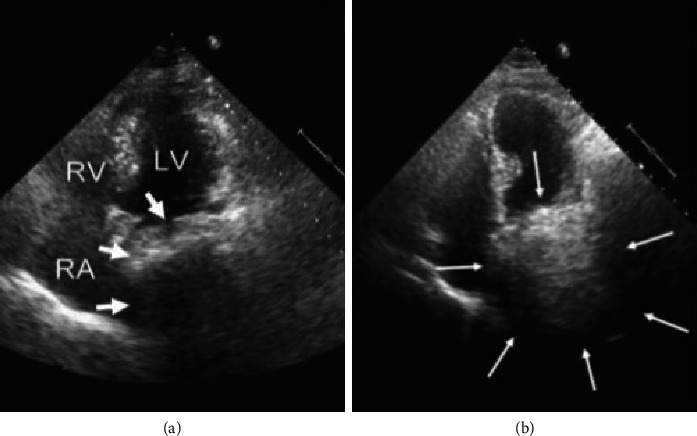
(a) Transthoracic echocardiography, apical 4-chamber view showing a mass of uncertain origin (intra-atrial vs. extracardiac) at left atrial posterior wall level (large arrows). (b) The same echocardiographic projection immediately after ingestion of a carbonated beverage showing the mass entirely filled with bubbles, thus confirming its extracardiac location (small arrows) (adapted from Bouzas-Mosquera et al.) [74]. Abbreviations: LV: left ventricle; RA: right atrium; RV: right ventricle.
